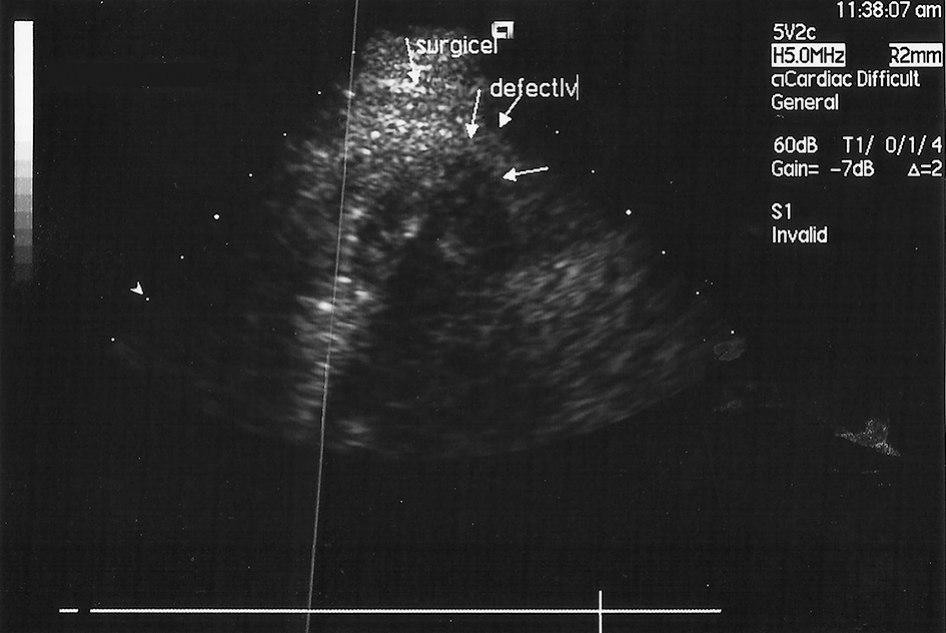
The Control Echocardiography Did not Reveal any Pericardial Effusion, and the Implanted Surgicel Was Documented as Well
